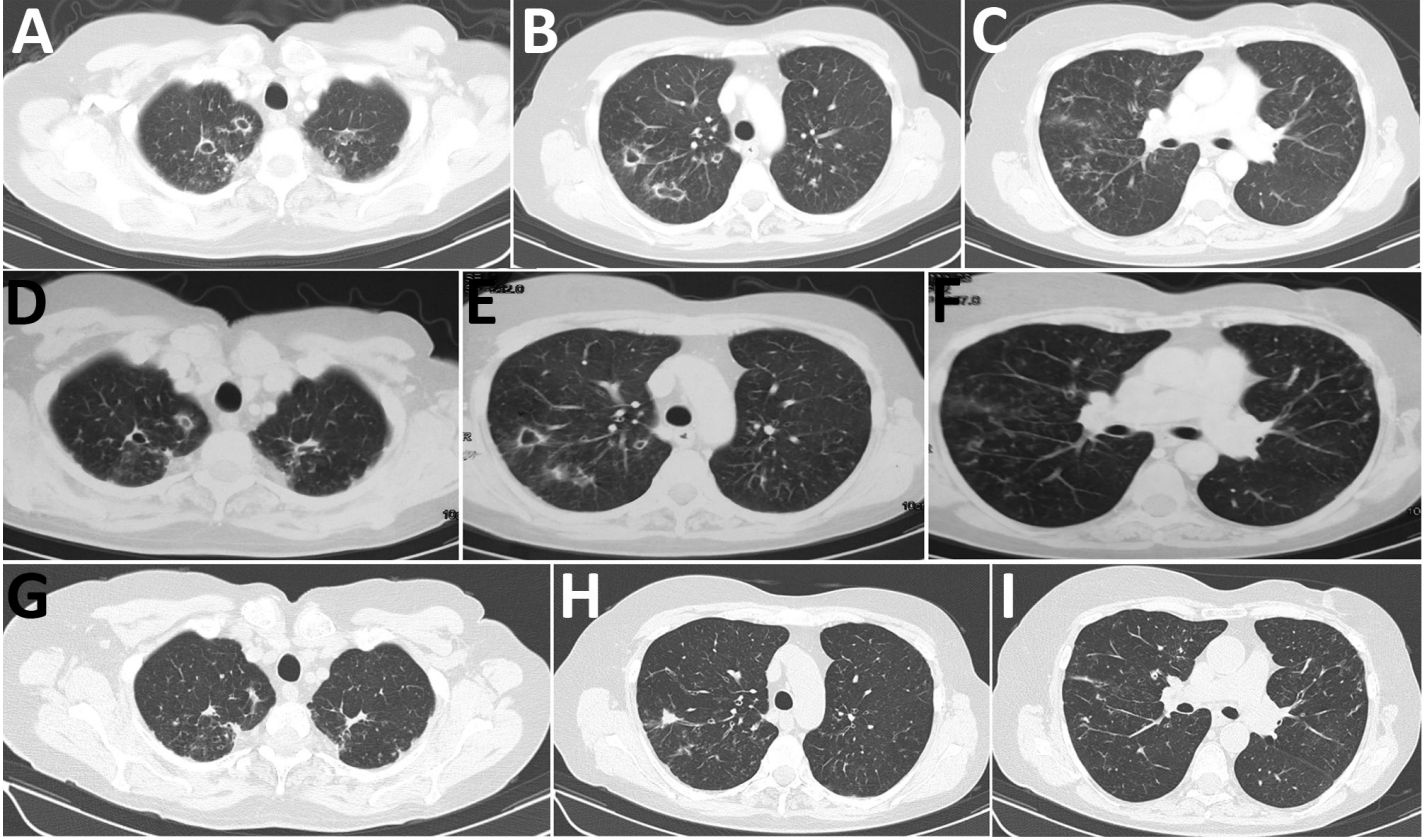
Chest computed tomography scan 1 month after antituberculosis treatment initiation (A–C), at the time of *Mycolicibacter kumamotonensis* identification (D–F), and 1 year after treatment initiation for *M. kumamotonensis* (G–I). Resolution of cavities and scar formation (A to D to G), resolution of pulmonary infiltrations (B to E to H), and hardening of the nodular appearances (C to F to I) are shown.
